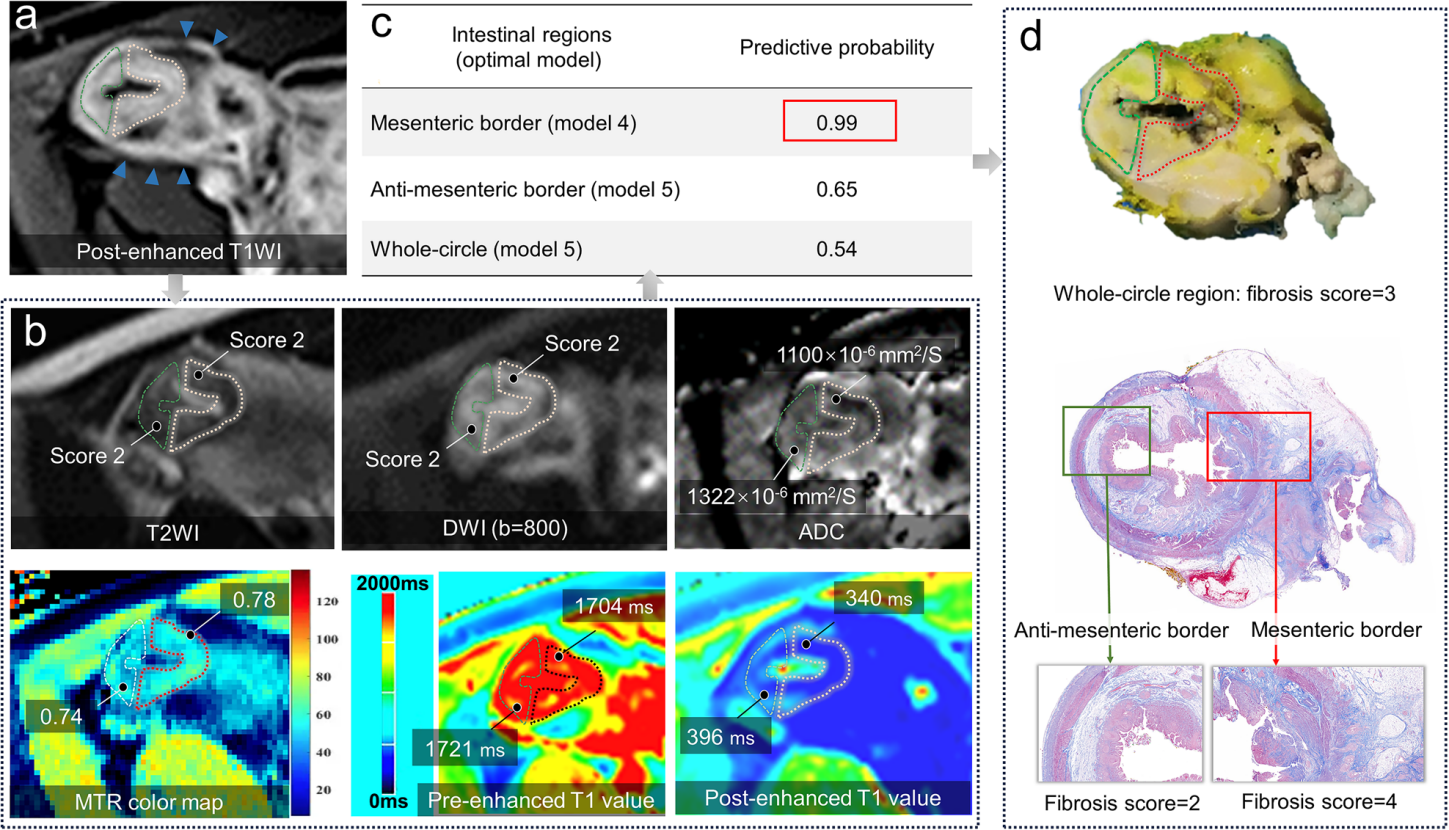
A representative case demonstrating the improved performance of MRE using a subregionalization diagnostic strategy. In the same patient as shown in Fig. 1, the division of mesenteric border (pink dotted line) and antimesenteric border (green dotted line) regions are performed according to MCFI (blue arrowheads) on axial post-enhanced T1WI (a) and are subsequently copied to other maps (b). The six MRE parameters in the whole-circle region are as follows: T2WI = 2, normalized MTR = 0.76, DWI = 2, ADC = 1311 × 10^-6^ mm^2^/S, pre-enhanced T1 value = 1,713 ms, post-enhanced T1 value = 395 ms. While their measurements in subregions are presented in the figures (b). The optimal multivariate MRE models corresponding to each region on APP are used for assessing fibrosis (c). Using pathological findings as a reference standard (d), the diagnostic accuracy of MRE improves significantly after subregionalization, particularly in the mesenteric border region (c). This diagnostic process, using an optimal MRE model in the mesenteric border region on APP, is also presented in Supplementary Fig. S5. MRE, Magnetic resonance enterography; MCFI, Mesenteric creeping fat index; T1WI, T1-weighted imaging; T2WI, T2-weighted imaging; DWI, Diffusion-weighted imaging; MTR, Magnetization transfer ratio; ADC, Apparent diffusion coefficient
